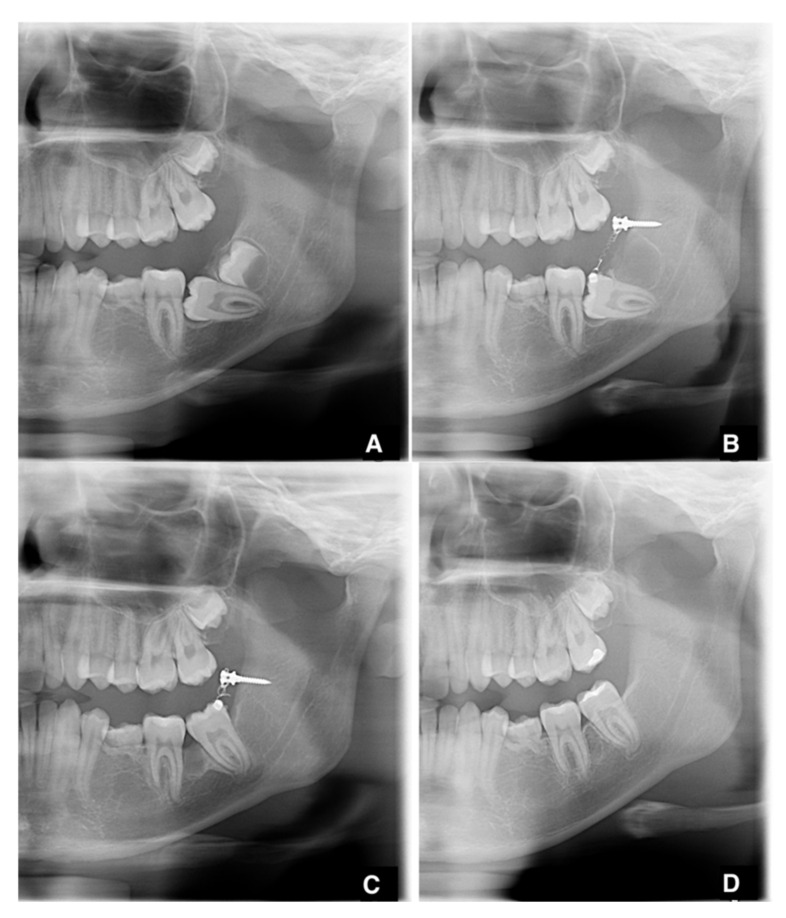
(A–D) The whole radiographic sequence of intervention (case 1).
